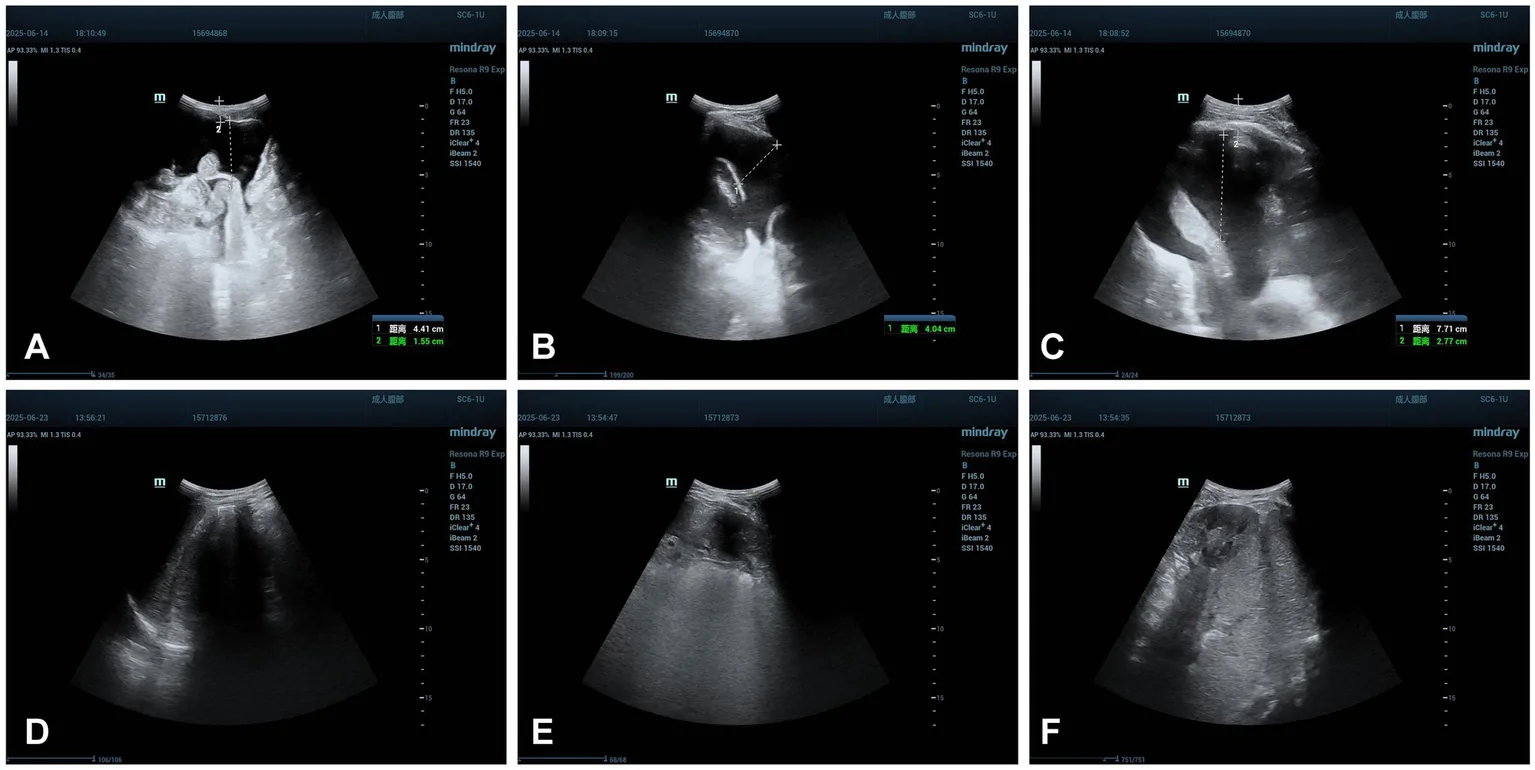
Ultrasonographic changes in abdominal and pleural effusions during hospitalization. (A) Abdominal ultrasonography on hospital day 7 showed ascites. (B,C) Thoracic ultrasonography on the same day showed left- and right-sided pleural effusions, respectively. (D) Follow-up abdominal ultrasonography on hospital day 15 showed marked resolution of ascites. (E,F) Follow-up thoracic ultrasonography on hospital day 15 showed no obvious residual pleural effusion bilaterally.
